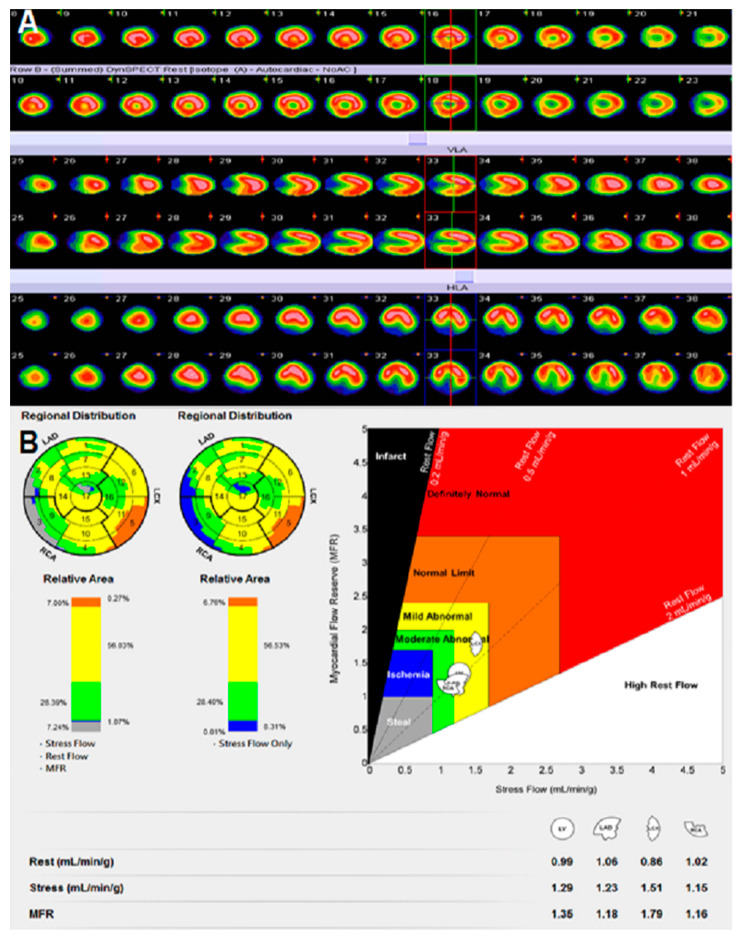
A 62 y/o male patient complained about chest pain and asked for help atthe CV OPD. He suffered from dyslipidemia, a risk factor for cardiovascular disease. He was referred for Dynamic SPECT/CT examination. Semi-quantitative MPI had no abnormal perfusion defect seen on (A). Quantitative coronary flow data of Dynamic SPECT/CT and CFC is shown on (B). CFC revealed moderate abnormal perfusion in RCA territory. Ischemic extent of LV was measured 8.3 (blue color), moderate abnormal perfusion of LV was measured as 28.4% (green color), and mild abnormal perfusion of LV was measured as 56% (yellow color). One month later, cardiac catheterization was arranged for him. Results of PCI were noted LCX-M 55% stenosis and RCA-orifice 70% stenosis. Due to this, the patient underwent PCI with stents over RCA territory. After PCI intervention, patient’s symptoms were improved and dyslipidemia was controlled with medication.
